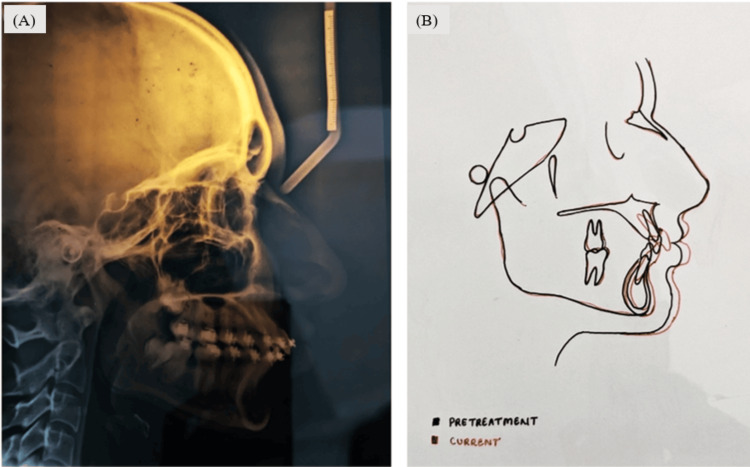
Post-treatment radiographic evaluation: (A) postoperative cephalogram, (B) superimposition of pretreatment and postoperative cephalogram
